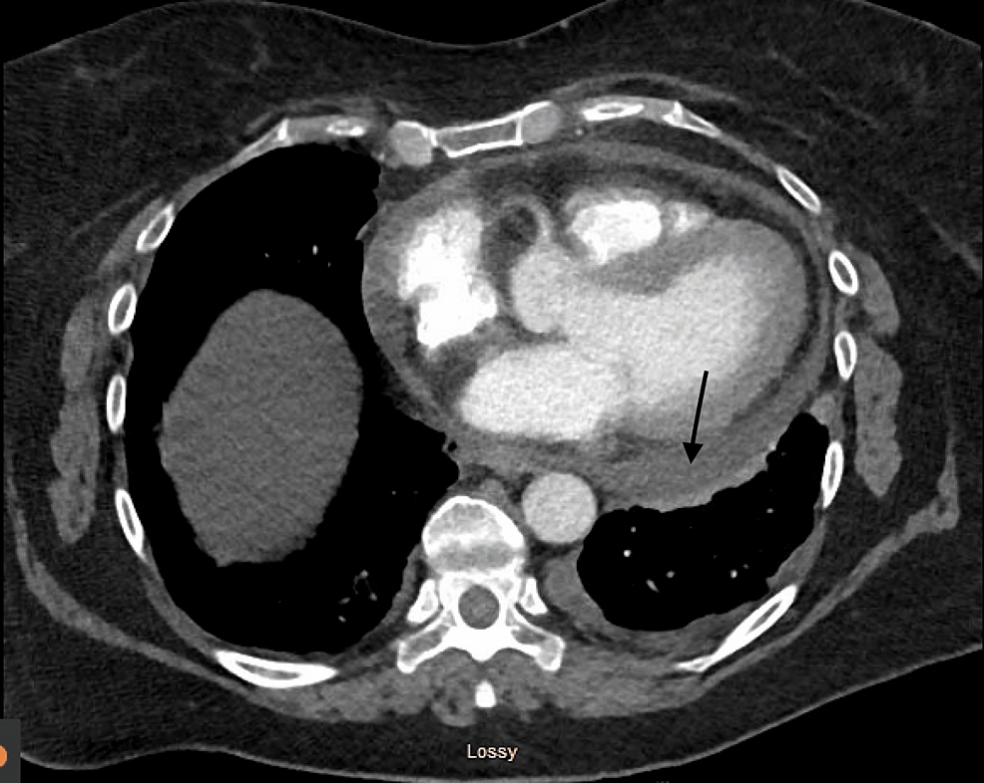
CTA chest with mild to moderate sized pericardial effusion CTA: computed tomography angiography
